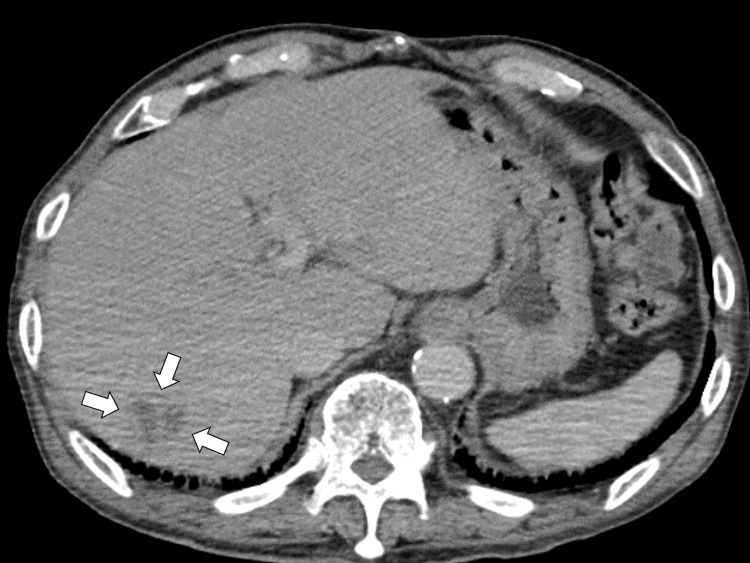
A dynamic contrast-enhanced abdominal computed tomography scan showing irregularly attenuated areas in the liver, consistent with hepatic abscesses (white arrows).
